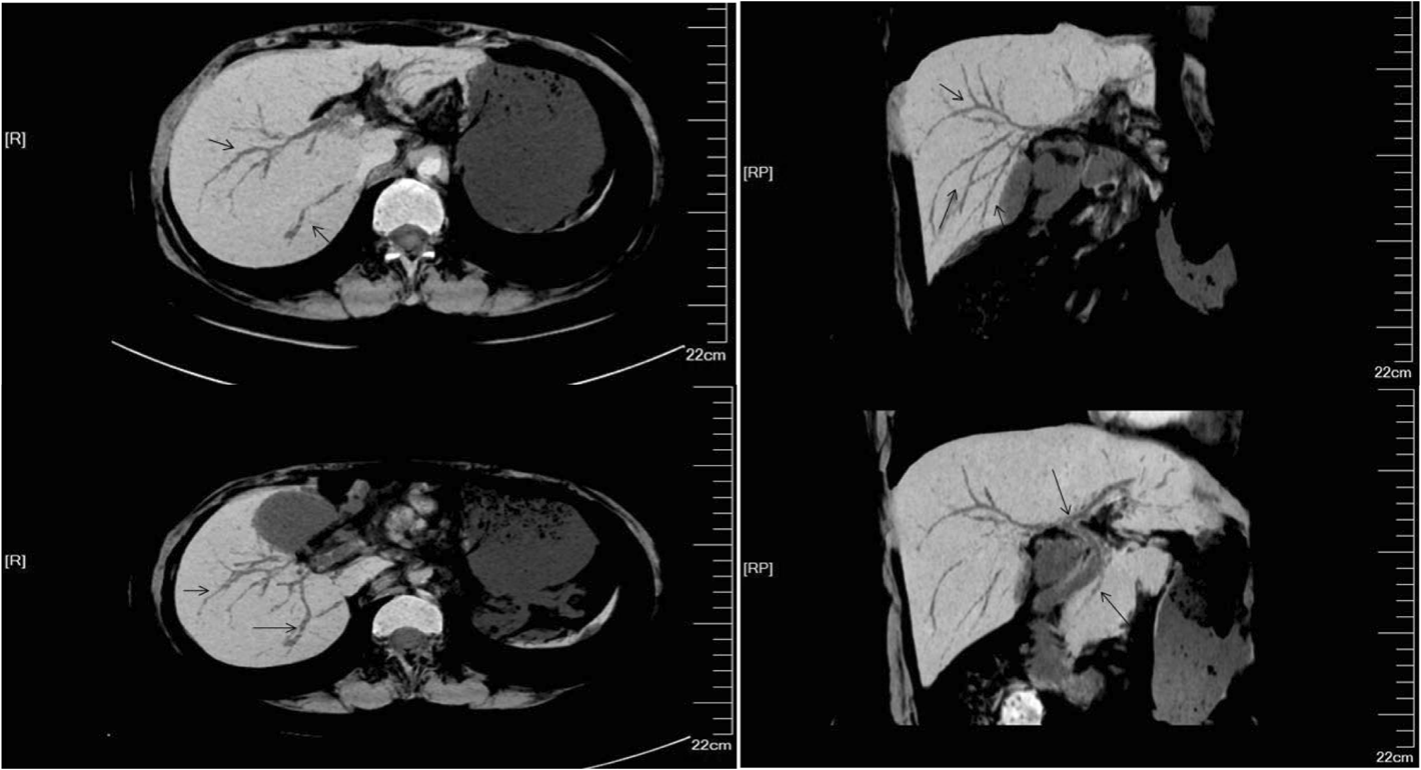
Dilation of the intrahepatic ducts by CT scan
